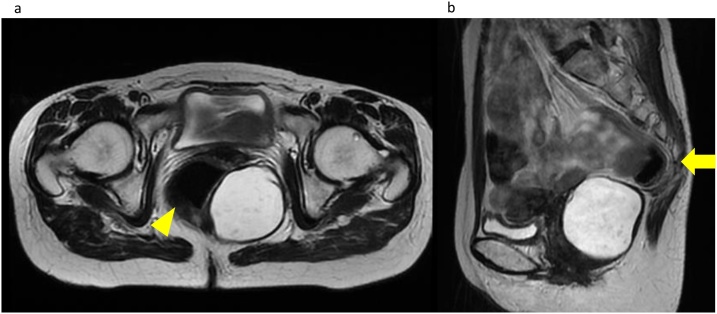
Magnetic resonance imaging shows a unilocular cystic tumor with a high signal intensity on T2-weighted images in the pelvis. (a) A well-defined homogeneous cystic mass is located in the left dorsal side of the rectum (arrow head) and (b) ventral side of the coccyx (arrow).
